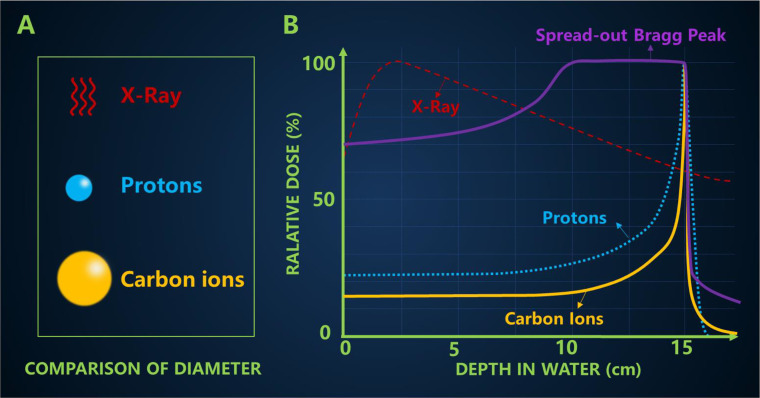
(A) Comparison of particle size (B) The typical depth dose curves of X-ray, protons and carbon ion beams, and a spread-out Bragg peak (SOBP) created by super-positioning many Bragg peaks at different depths.
